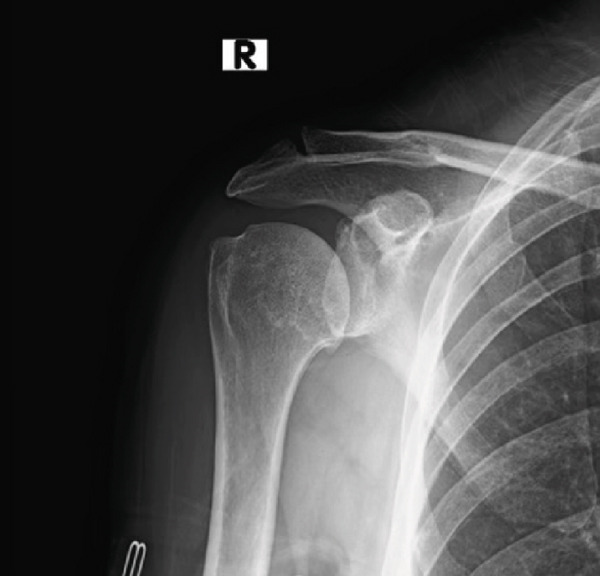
Preoperative shoulder radiograph showing joint space narrowing and osteophyte formation (August 17, 2022).
